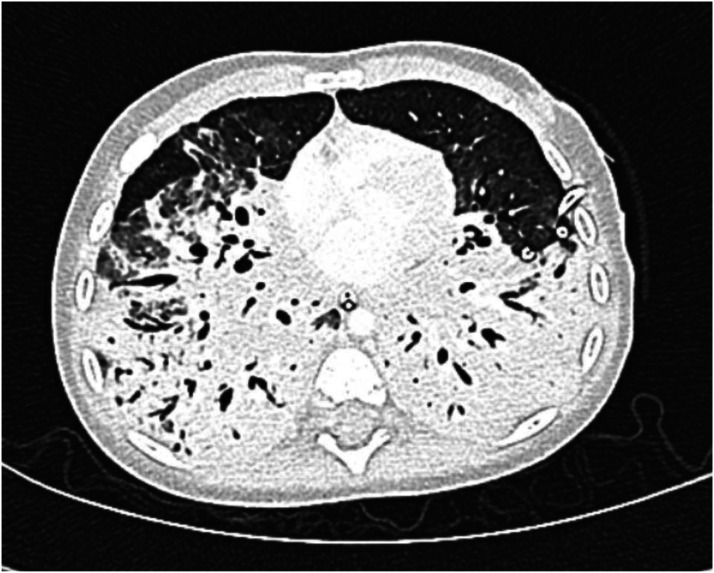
Chest CT on hospital day 11 demonstrating bilateral lower lobe lung necrosis.
